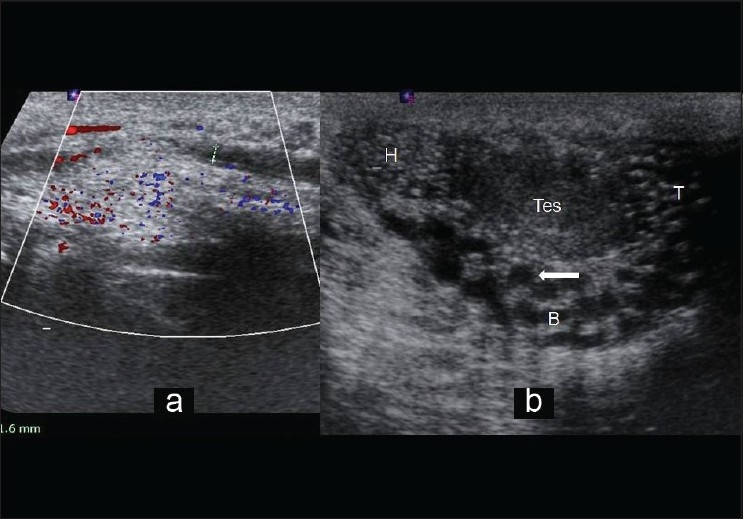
(a) High-frequency ultrasound longitudinal image of right spermatic cord showing dilatation of the right vas deferens in the region of right inguinal canal. (b) High-frequency ultrasound longitudinal image of right scrotal sac showing dilated seminiferous tubules along the complete length of epididymis (arrow). H, head; B, body; T, tail of epididymis; Tes, right testis
